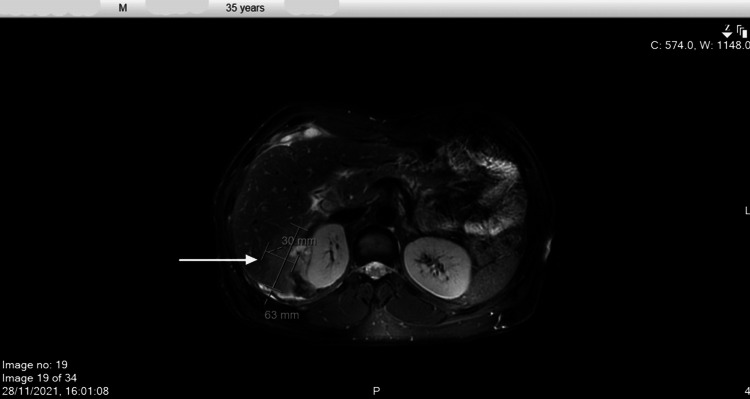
MRI abdomen/pelvic (axial plane) The image shows multifocal infiltrative lesions centered in the hepatorenal space invading the adjacent liver, right kidney, and abdominal wall. MRI, magnetic resonance imaging.
